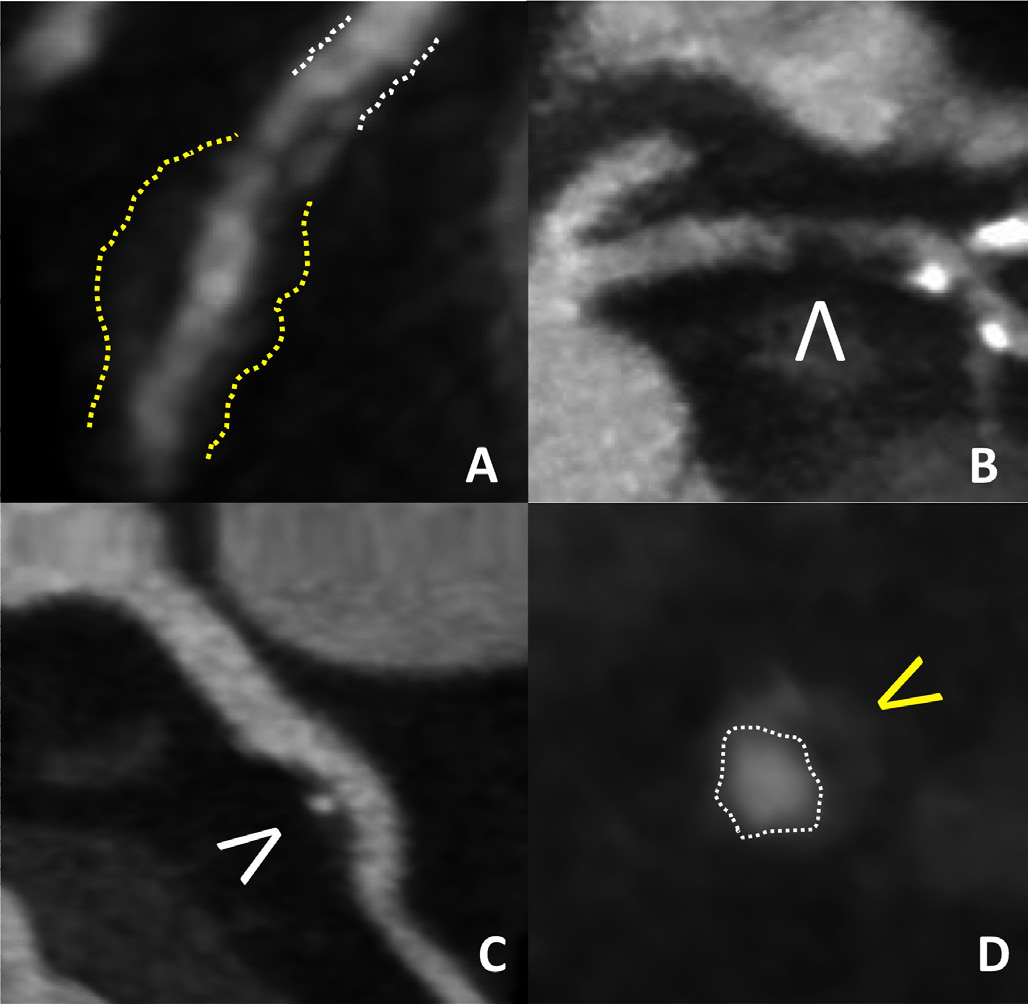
High-risk plaque features on CCTA. (A) Positive (Glagov) remodelling of plaque (yellow lines) maintains the coronary lumen (white lines) by outwardly remodelling atheromatous wall of the vessel. (B) Low-density non-calcified plaque (<30 HU) (white arrowhead). (C) Spotty calcification (white arrowhead. (D) Napkin ring sign (lumen—white line; plaque—yellow arrowhead). CCTA, coronary CT angiography.
